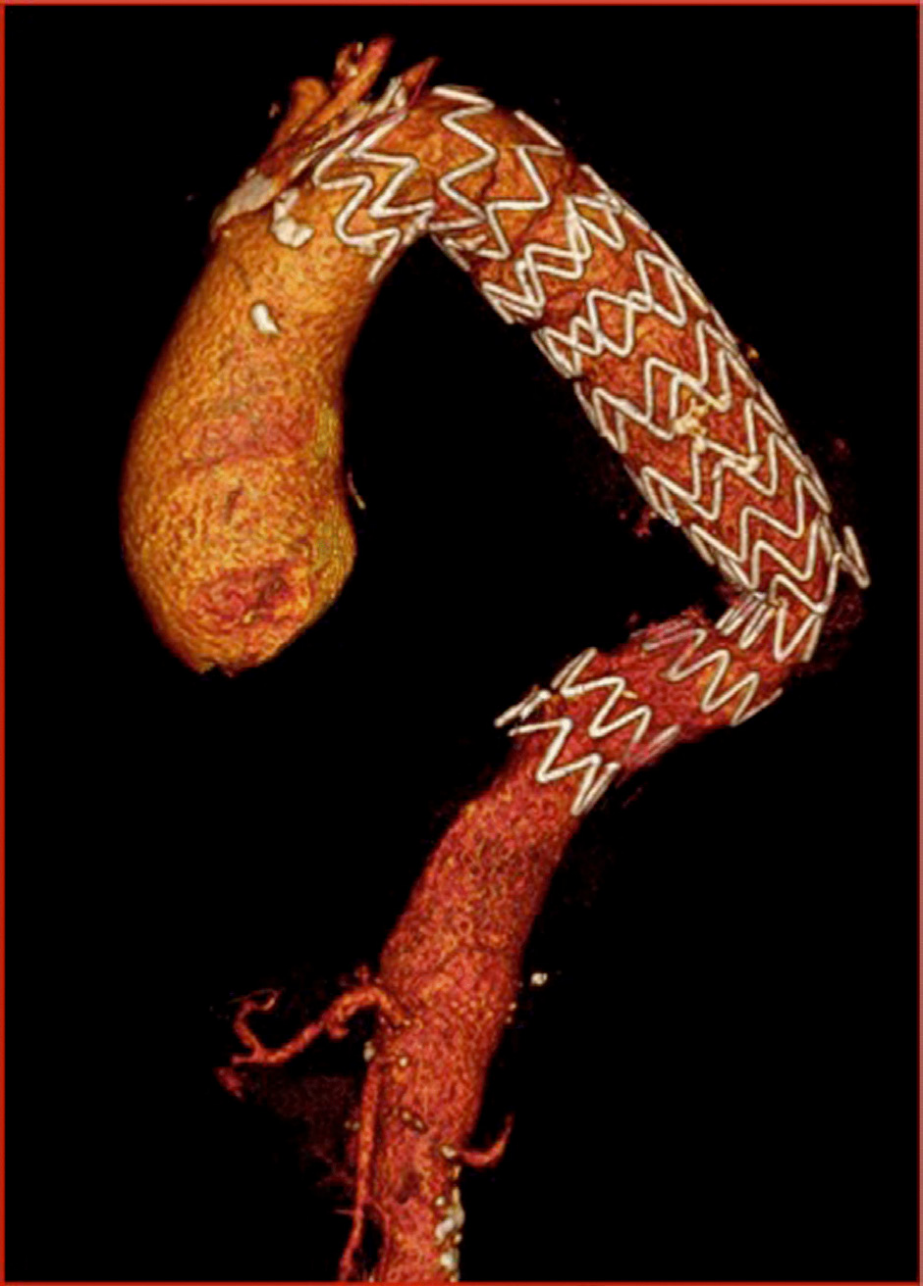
Postoperative CT scan showing correctly positioned endograft.
